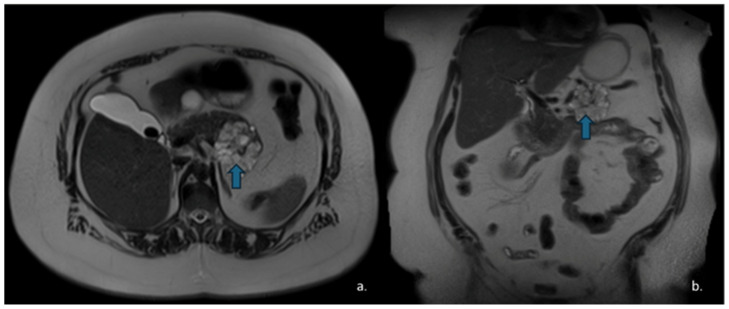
Axial (a) and coronal (b) T2-weighted HASTE sequences show a hyperintense lesion (arrow) located at the head–body passage of the pancreas. The lesion is characterized by thin, hypointense internal septa. Endoscopic ultrasound revealed a microcystic serous cystadenoma.
